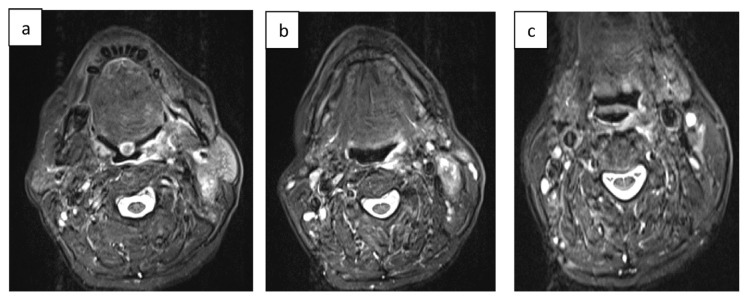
CT coronal images post-chemotherapy. a) CT image with a coronal cut on the tongue dorsum plane; b) CT image with a coronal cut on the mandibular plane; c) CT image with a coronal cut on the hyoid bone plane.
